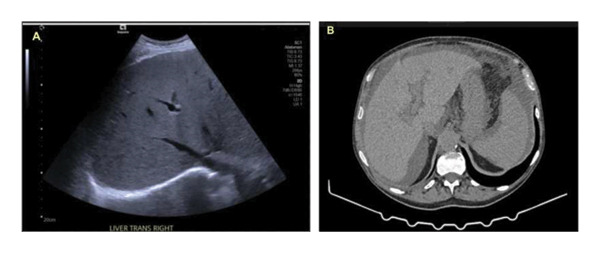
(A) Ultrasound showing increased background echogenicity of the liver. (B) Quad‐phase CT noncontrast liver demonstrating mild increased density of the liver compared with the spleen.
